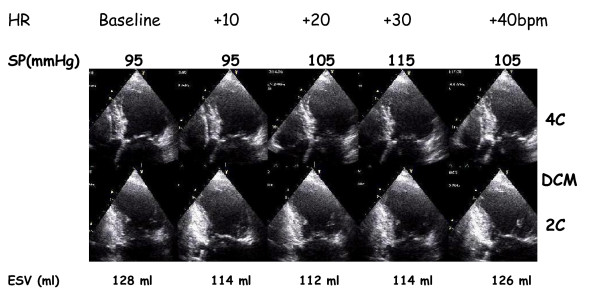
Methodology of the force-frequency curve with pacemaker stress echo in subject with post MI dilated cardiomyopathy and depressed baseline left ventricular function (EF = 30%). On the left, from upper to lower rows: heart rate from external programming of permanent pacemaker (first row); systolic blood pressure by cuff sphygmomanometar (second row); left ventricular end-systolic apical four (4C) and the two (2C) chamber view (third and fourth row); end-systolic volume calculated with biplane Simpson method (fifth row). An increased heart rate at peak pacing stress is accompanied by no change in end-systolic volumes (abnormal flat-biphasic FFR).
